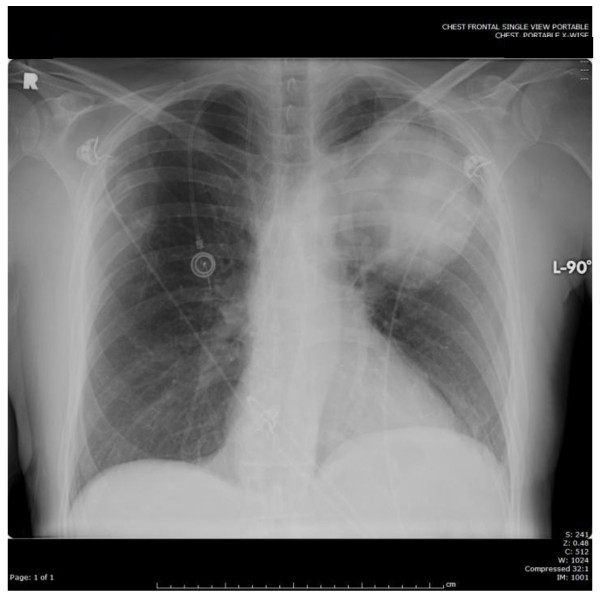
Por Chest X-ray showing the left upper lobe lung mass.
